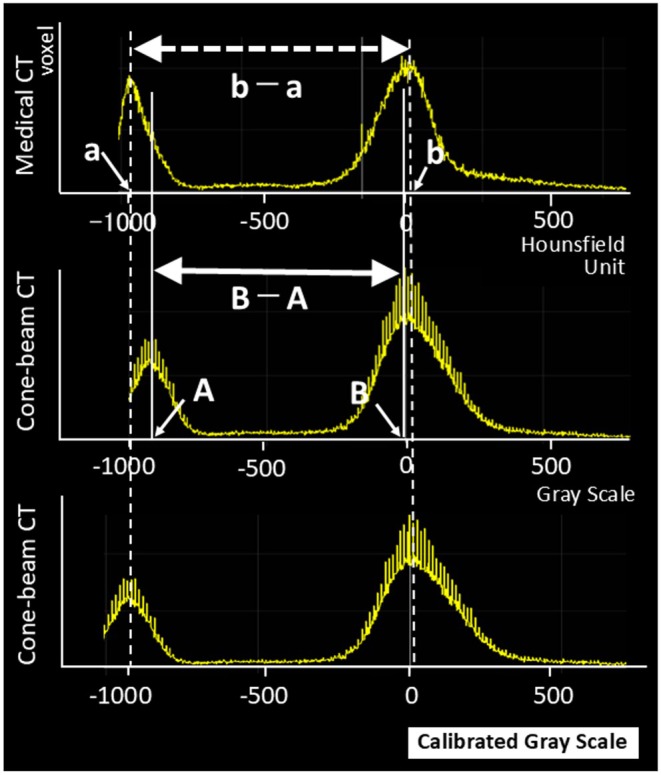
Calculation of cone‐beam computed tomography (CBCT) grey scale (GS) values corresponding to the medical CT (MDCT) Hounsfield unit (HU) Values. Top: Histogram peaks for the air and facial regions in MDCT (Table A1). Histogram peak corresponding to the air regions in MDCT (−999.4 ± 4.4 HU), b; Histogram peak for facial soft tissue in MDCT (36.3 ± 2.8 HU), b‐a; Distance between peaks for the air and facial soft tissue regions (−1035.7 ± 5.1 HU). Middle: Histogram peaks for air and facial soft tissue regions in CBCT (Appendix 1). A; Histogram peak corresponding to the air region in CBCT (−927.9 ± 4.0 GS), B; Histogram peak of the facial soft tissue region in CBCT (36.8 ± 5.1 GS), B‐A; Distance between the peaks of the air region and facial soft tissue region (−964.7 ± 6.7 GS). Lower row: CBCT GS values calibrated to correspond to the MDCT HU values. The distance between the CBCT histogram peaks was adjusted to match the distance between the air region histogram peak and the facial soft tissue histogram peak in MDCT. Using this unified scale, CBCT GS values corresponding to MDCT HU values indicating muscle and fat were calculated.
